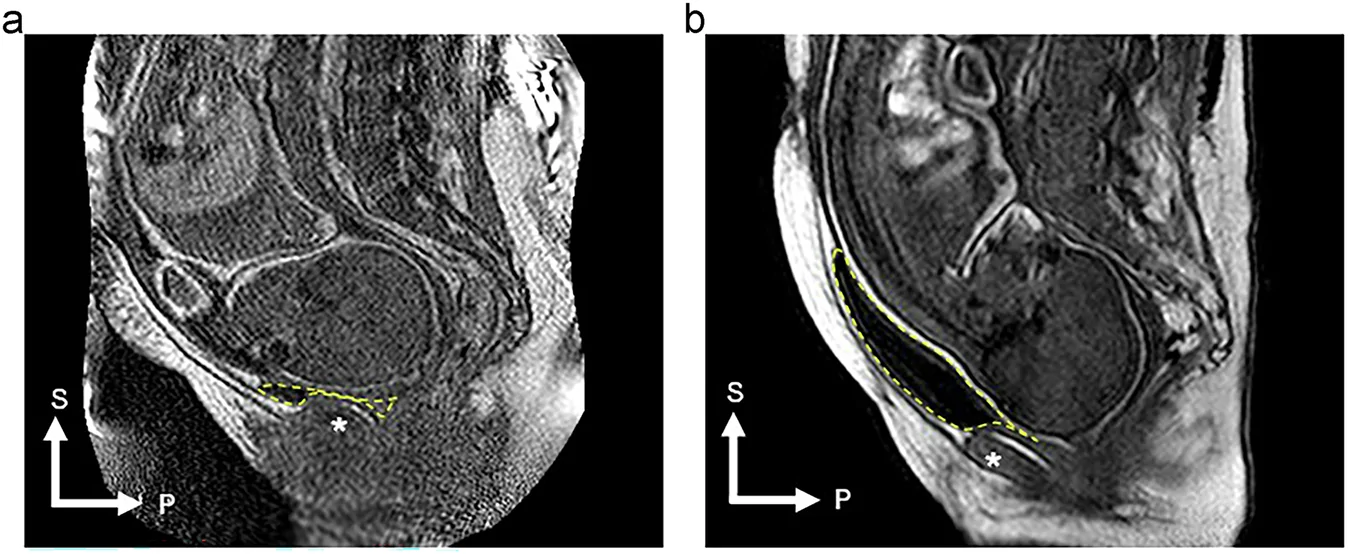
Sagittal MRI of the bladder (yellow dotted line) during the SSL when empty (a) or partially filled (b). When it is empty, the bladder is compressed between the pubis (*) and the fetal head. The downward movement of the fetus places a shearing force on the bladder and its supporting structures. The partially filled bladder is above the pubic symphysis and posterior to the lower abdominal musculature. In this position, it appears to avoid most of the forces related to fetal expulsion and childbirth. MRI, Magnetic resonance imaging; SSL, Second stage of labor
